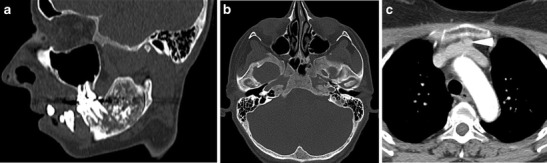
a) Sagittal oblique CT and b) axial CT image of the skull base c) axial CT upper thorax. 34-yr-old female with undiagnosed HPT-JT who underwent a CT to assess a left mandibular ramus lesion (a) subsequently proven to be an ossifying fibroma. She was incidentally noted to have generalised bony sclerosis (b) suggestive of hyperparathyroid bone disease and further imaging (c) revealed a retrosternal parathyroid adenoma (arrowhead) anterior to the left brachiocephalic veinIn HPT-JT, parathyroid adenomas develop in a younger population with a mean age of diagnosis at 33 years, and there is a propensity for them to reoccur following removal as well as developing new adenomas [2]. In contrast, sporadic parathyroid adenomas typically occur as solitary single episode tumours beyond 40 years of age, with a predilection for the female population [9]. HPT-JT individuals are at a higher risk of developing parathyroid carcinoma, a rare malignancy in the general population [10].Parathyroid adenomas and carcinomas appear as solitary solid soft tissue masses the majority of which are juxtathyroid and located immediately posterior or inferior to the thyroid gland, although they can be located as inferior as the mediastinum [11]. First line assessment to identify parathyroid gland enlargement varies between institutions, but is typically performed with a combination of ultrasound and nuclear medicine scintigraphy. On ultrasound, parathyroid adenomas and carcinomas are uniformly hypoechoic and often demonstrate increased vascularity with identification of a vascular pedicle (Fig. 9) [11]. Nuclear medicine ‘wash out’ scinitigraphy using Technetium 99 m Sestamibi tracer is the ‘gold standard’ for the detection of parathyroid adenoma [12]. The tracer is retained within parathyroid adenoma and carcinomas and demonstrates increased uptake on both early and late imaging (Fig. 10). In contrast, there is rapid wash out of tracer from the adjacent thyroid gland [13]. CT and MRI are employed when there is non-concordance between first line imaging investigations and in patients who have typically undergone previous surgical intervention to remove a parathyroid adenoma/carcinoma [11]. Selective venous PTH sampling can be used to localize a suspected occult parathyroid adenoma.
